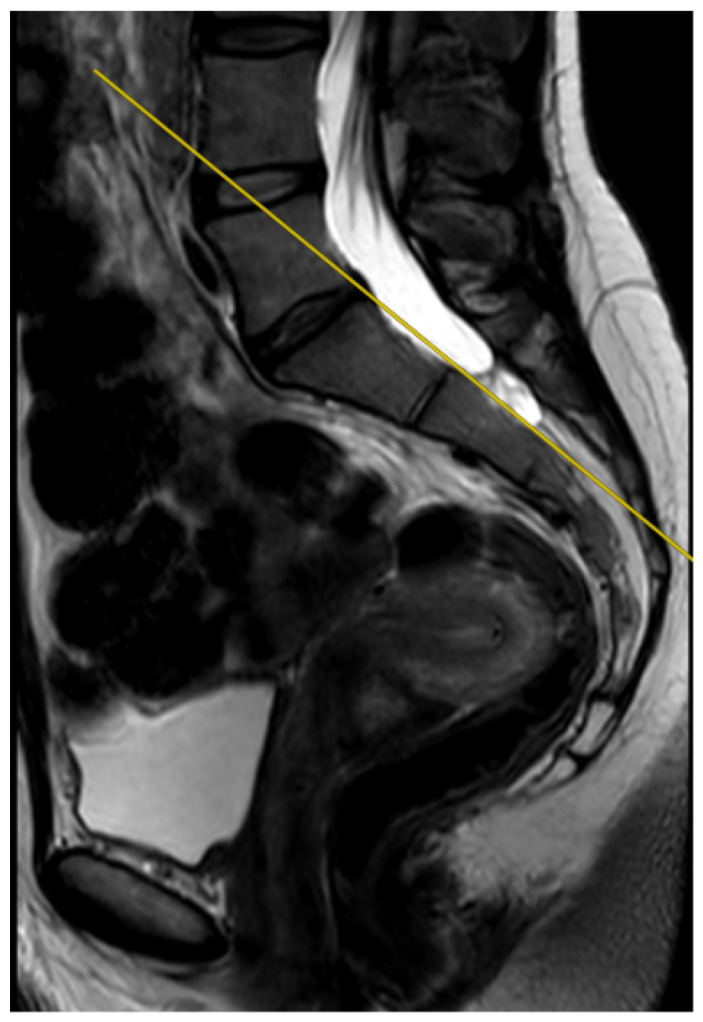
The measured deviation angle equals 0 degrees, properly performed MRI examination of the sacroiliac joints, T2-weighted sequence. The yellow line marks the actual plane in which the slices were acquired, while it is also the fully correct line drawn between the posterior edges of the S1 and S2 vertebrae.
